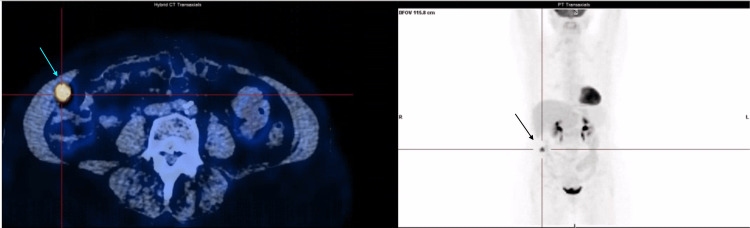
Positron emission tomography-computed tomography showing a solitary hypermetabolic lesion in the right anterolateral abdominal wall, consistent with isolated metastatic disease, with no evidence of additional hypermetabolic foci
